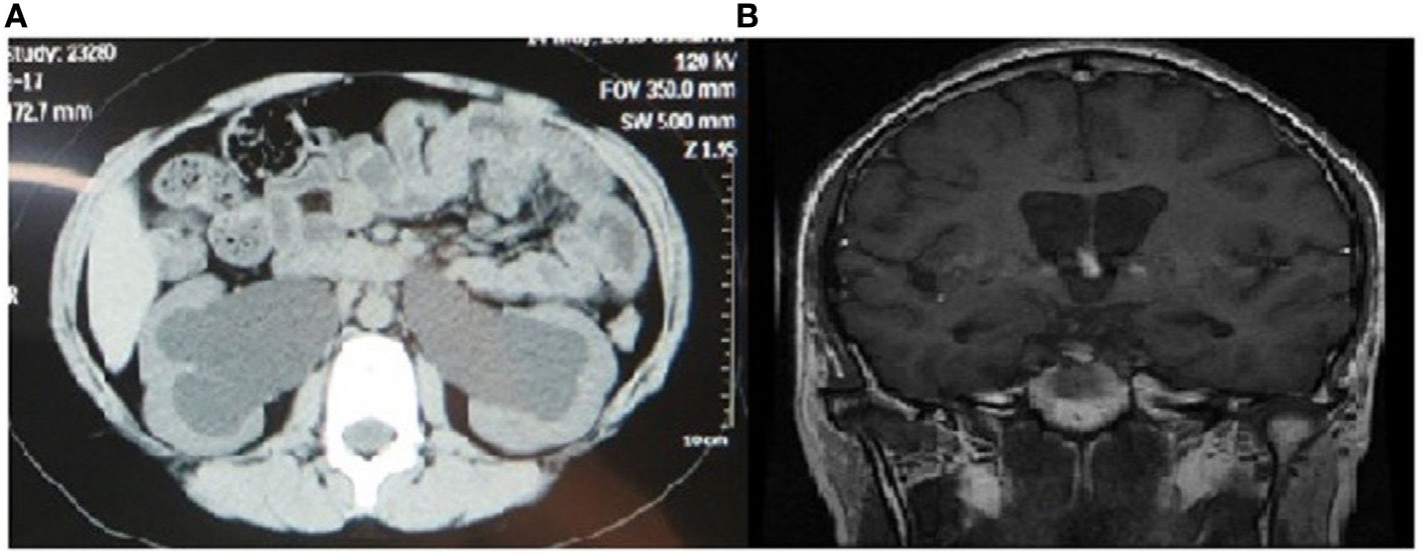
Image findings of urinary and nerve systems in patients with Wolfram syndrome. (A) Abdominal computed tomography (plain scan view) showing bilateral dilated renal pelvis of Case 3 in 10 years. (B) Brain magnetic resonance imaging (coronal/sagittal view) showing cerebral ventricle dilation, bulging corpus callosum, and hydrocephalus.
